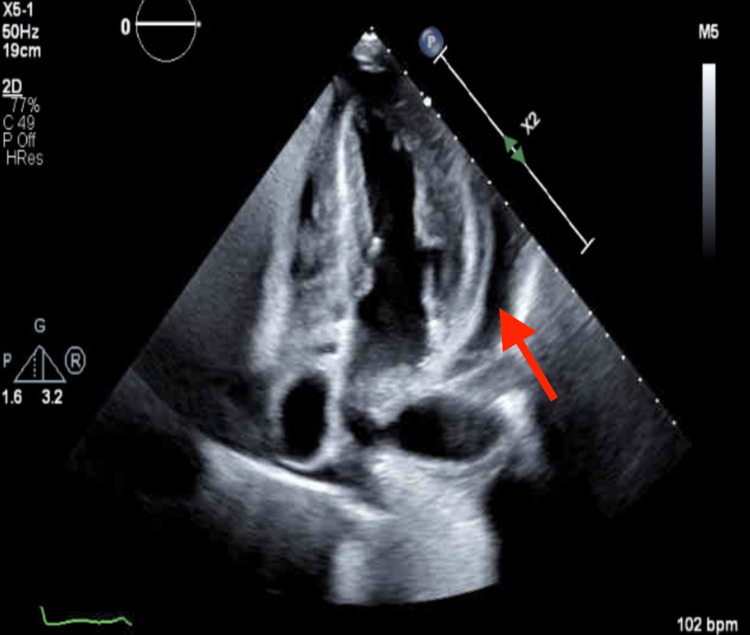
Transthoracic echocardiogram, parasternal long axis view, three weeks after the admission demonstrating stable large pericardial effusion at the LV posterior wall The red arrow points to the pericardial effusion.
